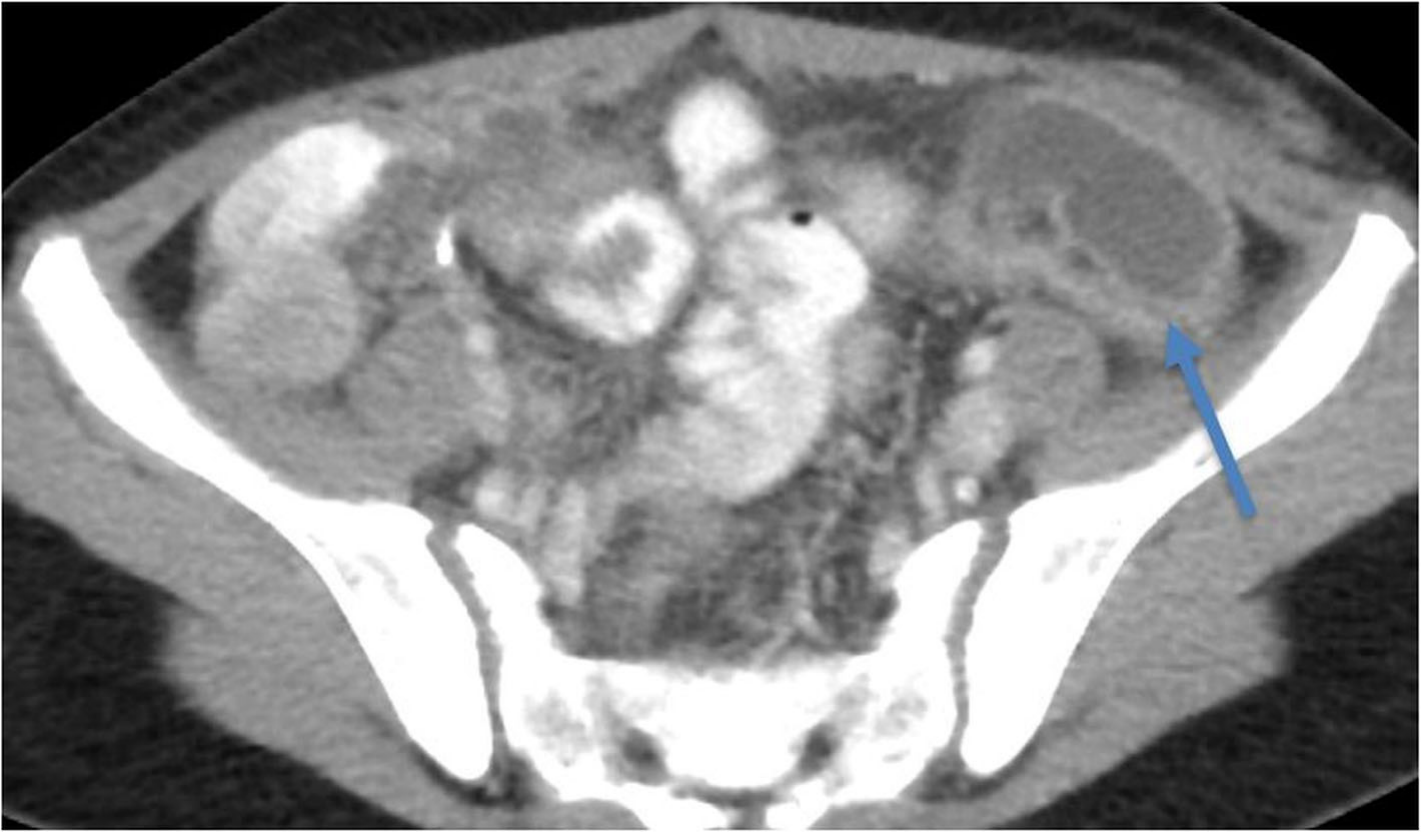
Transverse abdomen CT image of a 14 yrs. M (42 kg) acquired at 4.4 mGy. Interloop abscess (arrow) and overall image quality scored optimal (scores 3 or 4) by all readers
